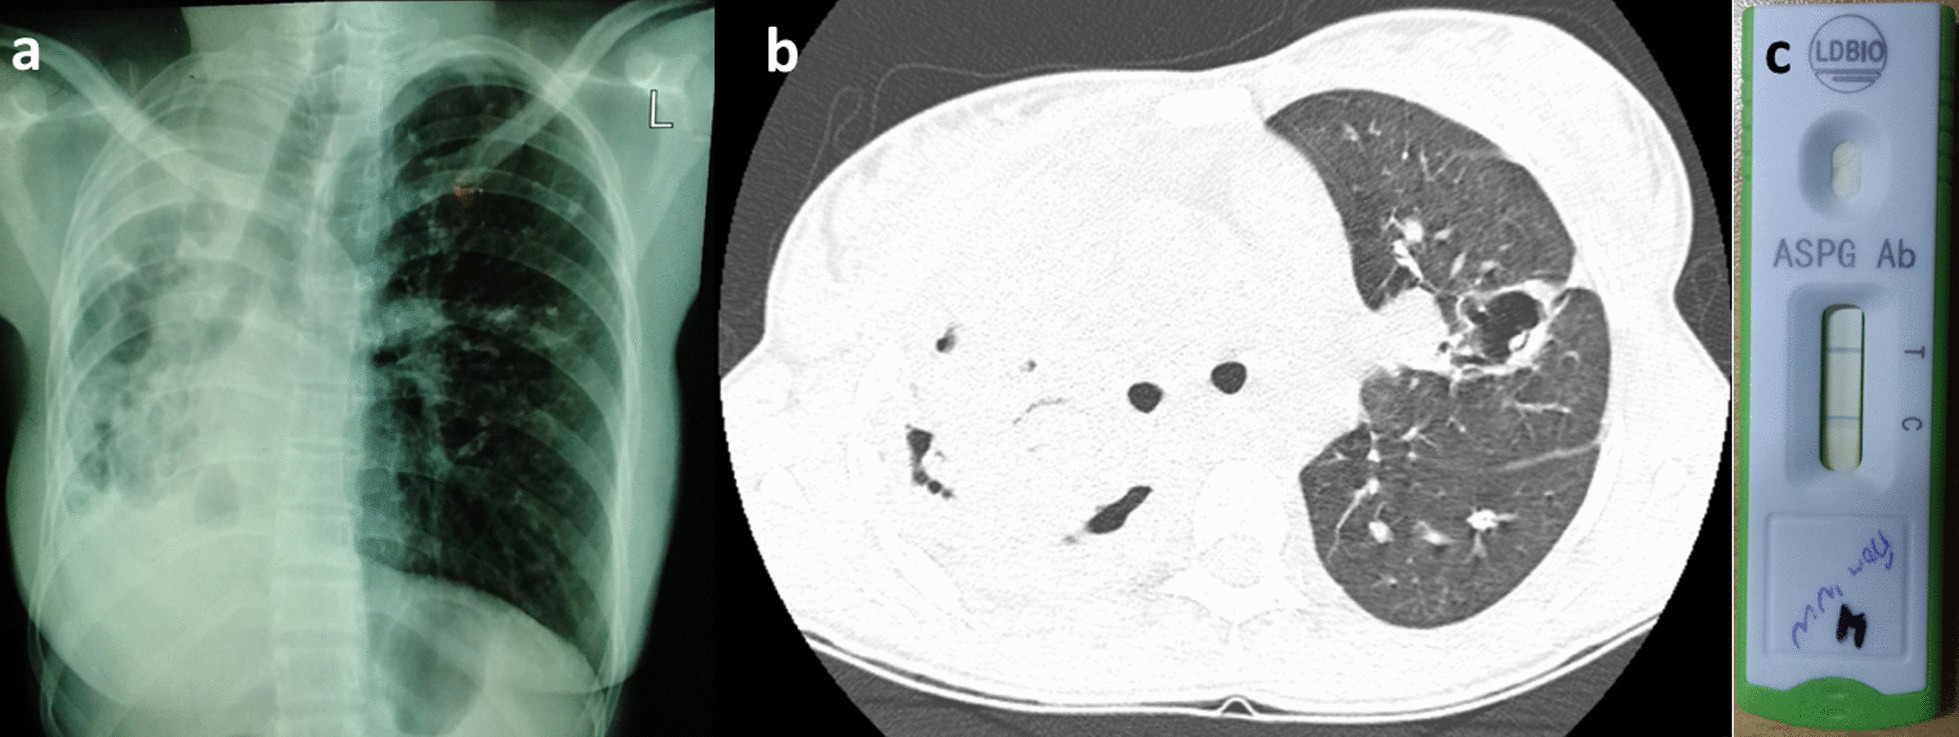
Case 3. a Chest x-ray showing post-inflammatory fibrotic changes in the right lung with features of bronchiectasis on the left with a left perihilar cavity. b Chest CT scan showing a thick-walled cavity with an intracavitary nodule and bronchiectasis. c Positive *Aspergillus* IgM-IgG LFD
